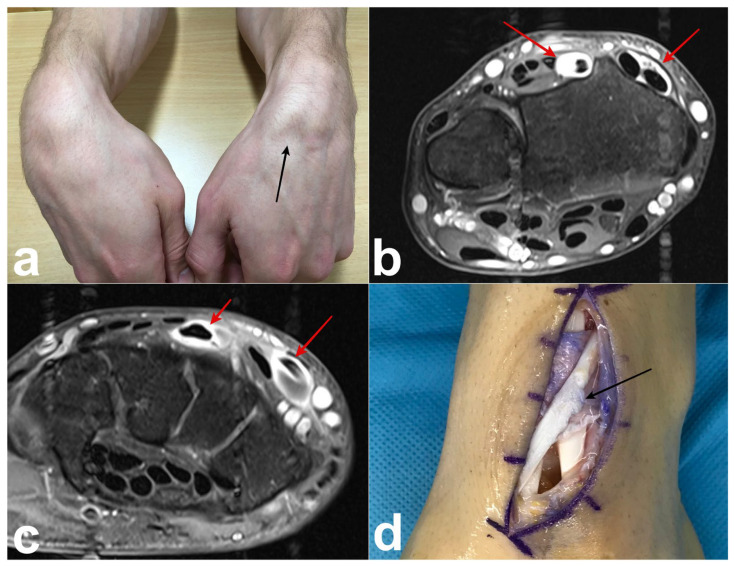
Case 3. (a) “heart-shaped” swelling (black arrow) of the dorso-radial aspect of the left wrist. (b,c) DIT: Tenosynovitis of the EPL, ECRB, and ECRL tendons (red arrows) of the left wrist on MRI (axial T2-weighted turbo spin-echo with fat saturation sequence). (d) The preoperative photograph after teno-synovectomy and the subcutaneous transposition of the EPL show evidence of tendinosis, with fraying on the superficial surface of the tendon.
